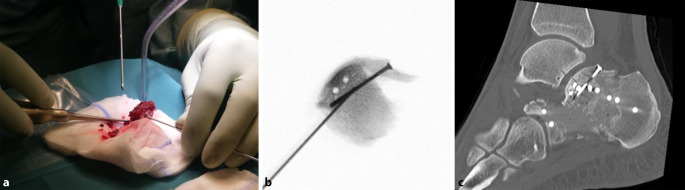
Ex-situ-Rekonstruktion der posterioren Gelenkfacette. a Intraoperative Situation, b situatives BV-Bild, c postoperatives CT
